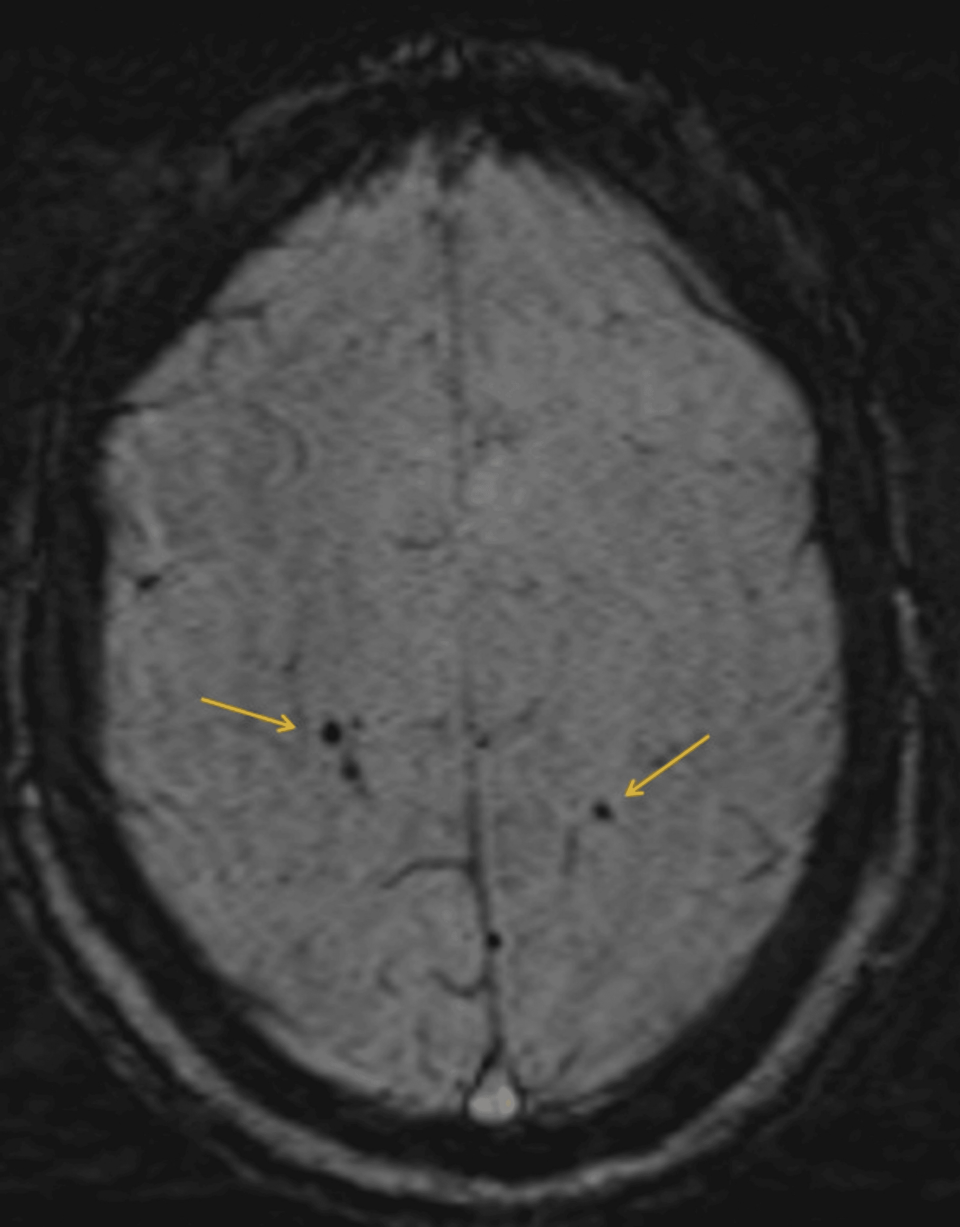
SWI on MRI of the head axial view demonstrates multiple corresponding focal areas of signal drop without a blooming artifact. No ischemic changes can be seen on the MRI. SWI: susceptibility-weighted imaging; MRI: magnetic resonance imaging
